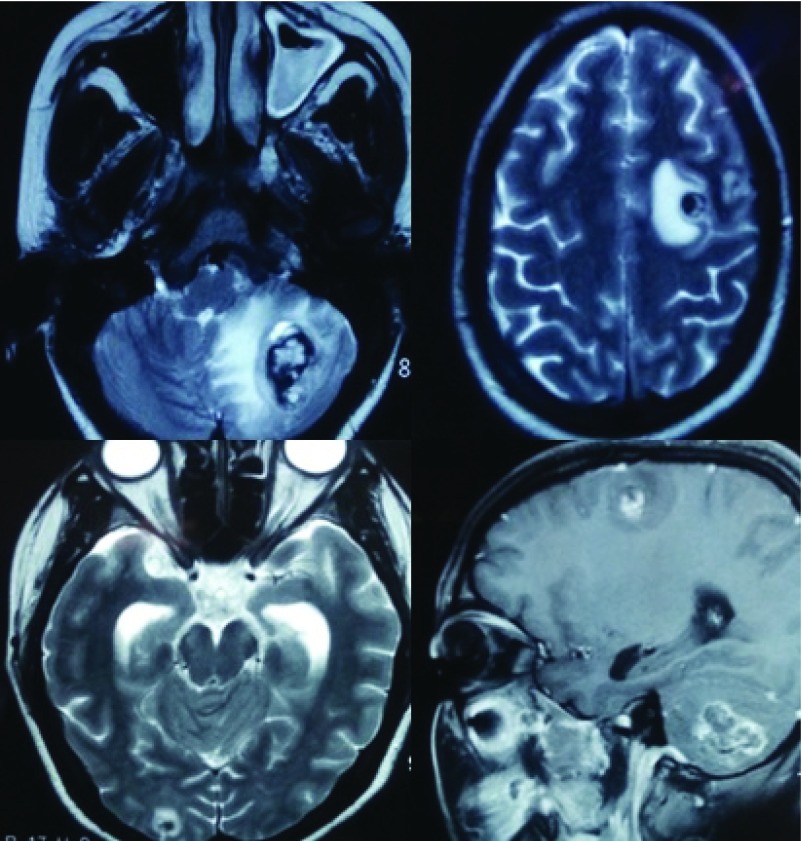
MRI images showing multiple lesions within the brain at different phase of resolution.
